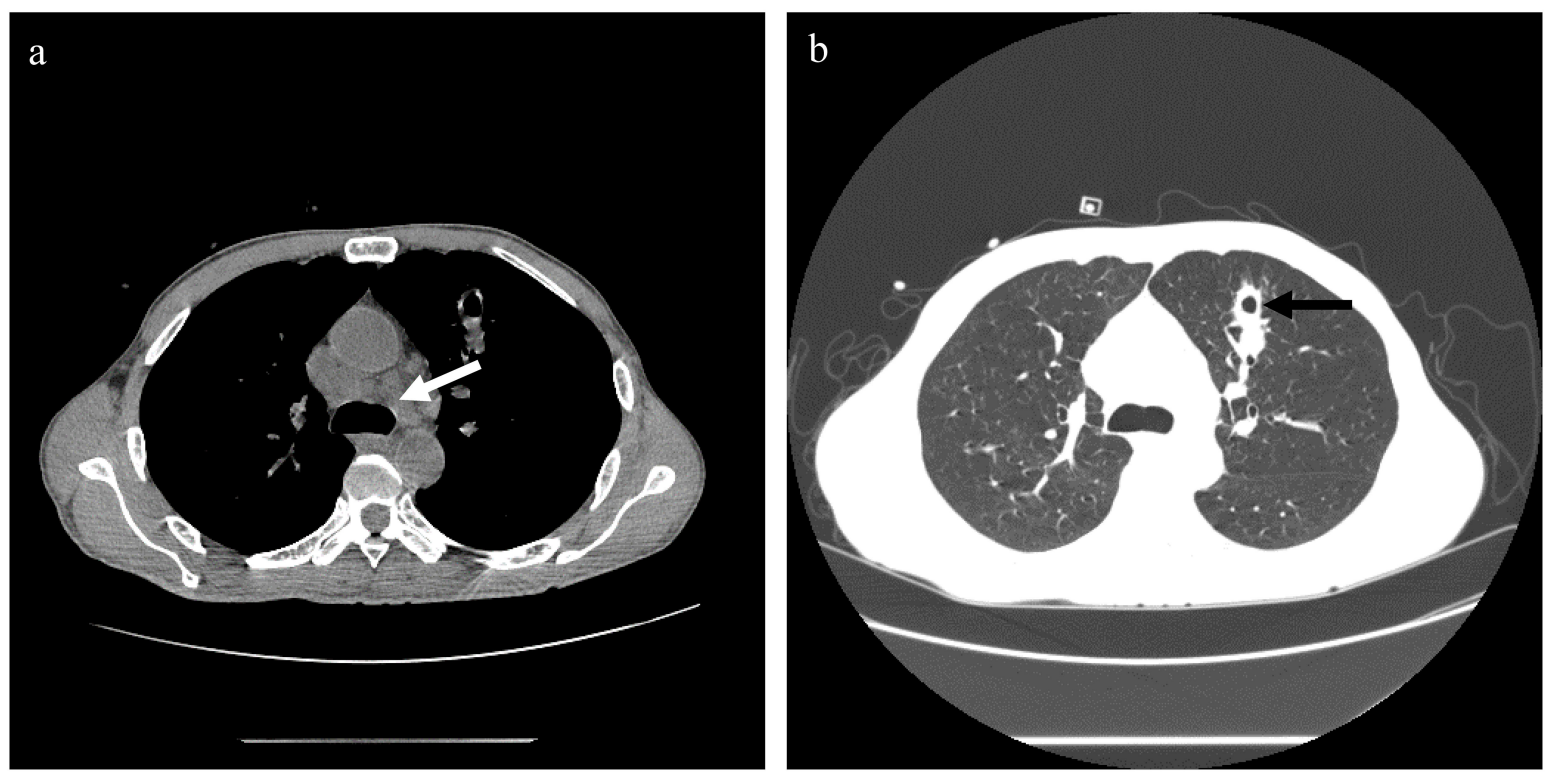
Chest CT scan of case 1. (A) The mediastinal window, showing multiple mediastinal lymph node enlargements (white arrow). (B) The lung window, showing uniform ground-glass exudation in both lungs. The left upper lung sees the irregular edge of the nodule (black arrow), and there is a cavity in the middle of the nodule. No obvious pleural effusion was found.
